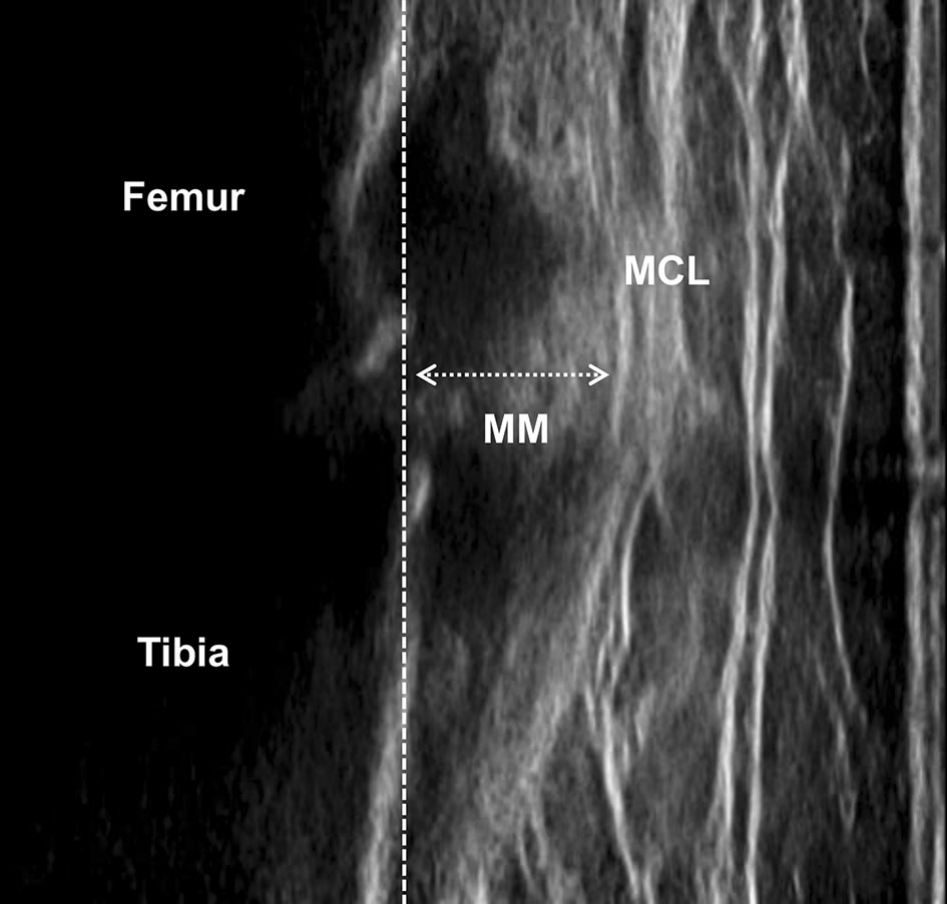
The evaluation of meniscus extrusion on ultrasound. *MM* medial meniscus, *MCL* medial collateral ligament. The dashed line shows the cortex of the medial tibial plateau. The extruded medial meniscus is shown as the dashed arrow line.
